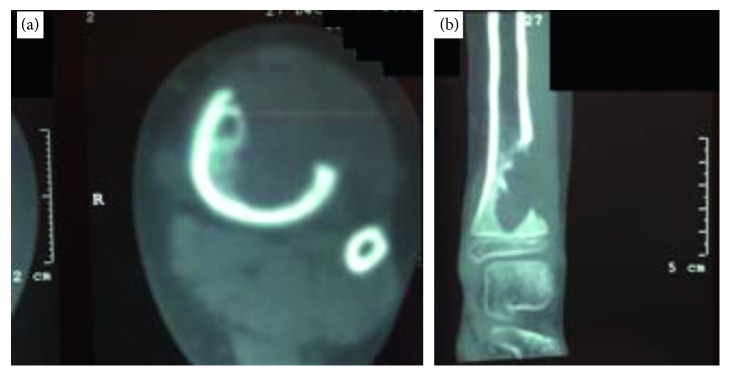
(a) Axial CT showing destruction of the anterior tibial cortex with extension into soft tissue. (b) Coronal CT showing ill-defined distal tibial lesion with cortical destruction.
